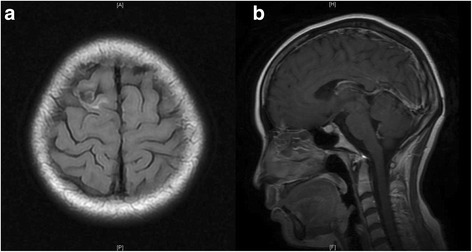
MRI scan of brain after albendazole treatment: a Axial view of T2 W flair. The lesion had obviously diminished after treatment. b Sagittal view of T1 W flair + C. The lesion had obviously diminished and was without enhancement
